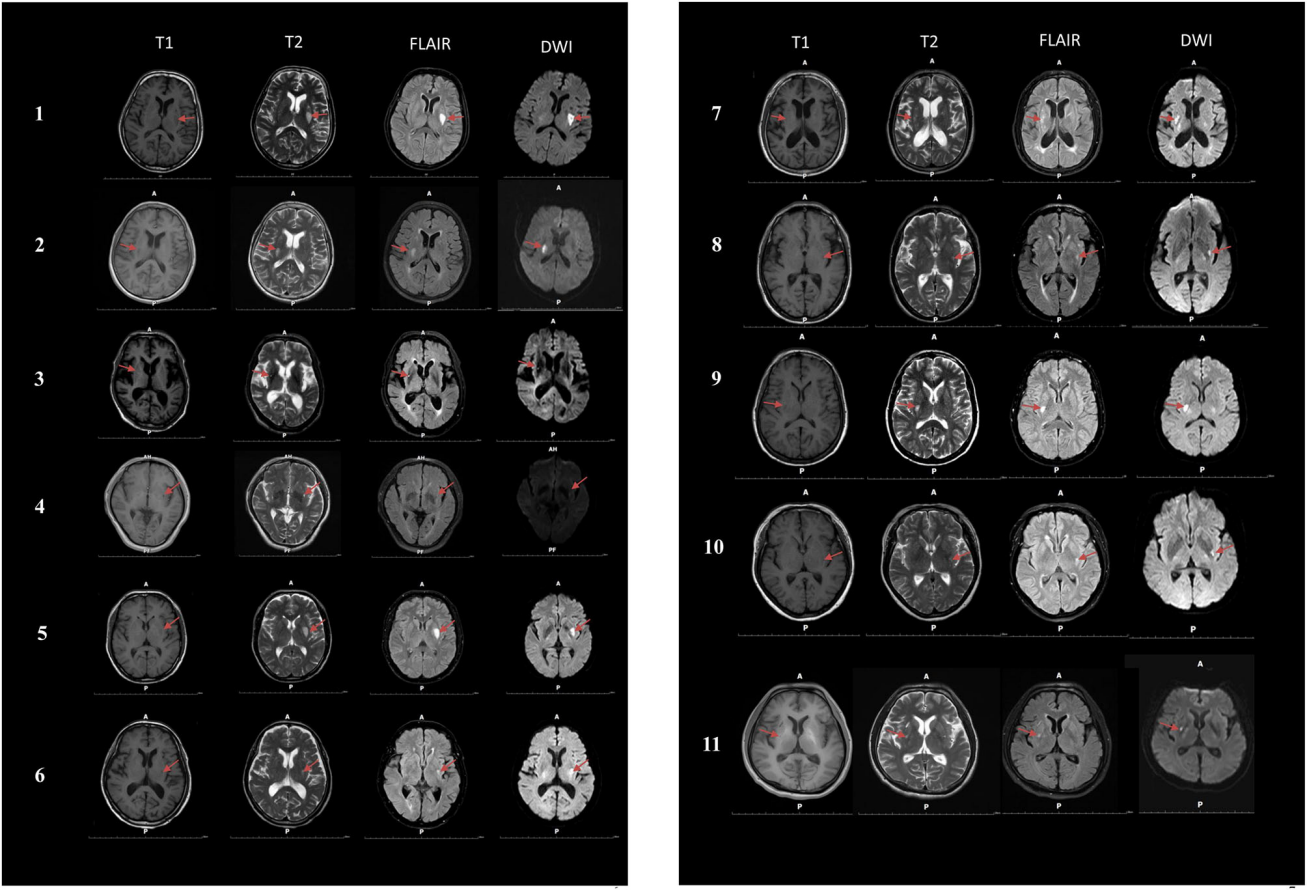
Magnetic resonance imaging (MRI) was performed on 11 smokers with dorsal striatum damage. T1, T1-weighted images; T2, T2-weighted images; FLAIR, Fluid attenuated inversion recovery; DWI, Diffusion weighted imaging.
